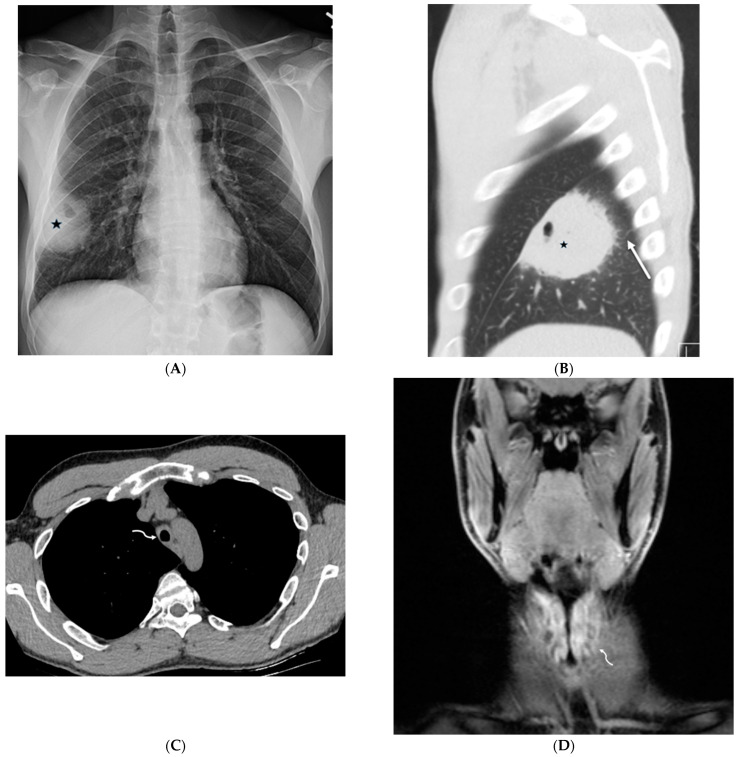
A 31-year-old male presenting with cough, intermittent nose bleeds, hoarseness, and a sensation of clogged ears. A frontal chest radiograph (A) demonstrates a cavitary mass (star) in the right lower lobe. A sagittal chest CT image (B) shows a cavitary mass (star) with surrounding interlobular septal thickening (solid white arrow). An axial chest CT image (C) and coronal neck fat-suppressed T1-weighted contrast-enhanced MR image (D) show marked luminal narrowing of trachea and subglottic larynx (curved white arrow) with circumferential soft tissue. A lung biopsy revealed multinucleated giant cells consistent with a diagnosis of granulomatosis with polyangiitis (GPA).
